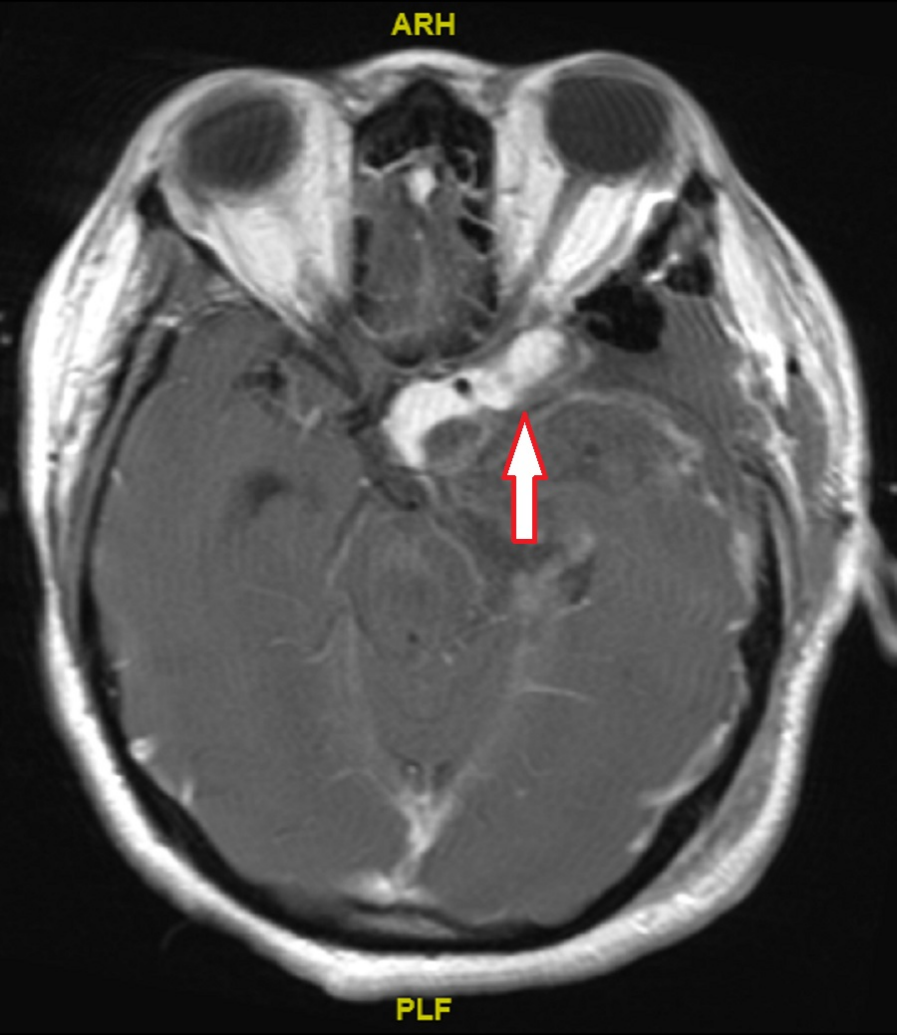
Post-surgery T1 Contrast-enhanced Magnetic Resonance Imaging Axial view of tumour, demonstrating residual tumour after surgery (arrow)
